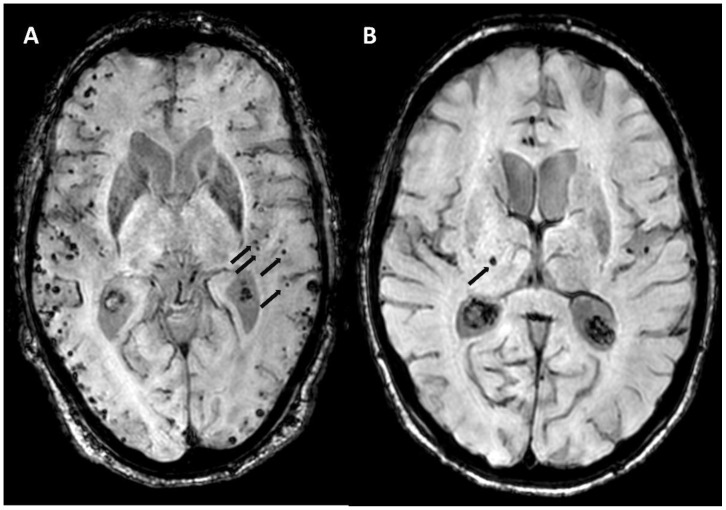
(A) Multiple lobar cerebral microbleeds (black arrows; not all microbleeds are marked). (B) Single deep-seated thalamic CMB (black arrow) on venBOLD MRI (3T, TE = 25 ms, TR = 18 ms, slice thickness 1 mm).
